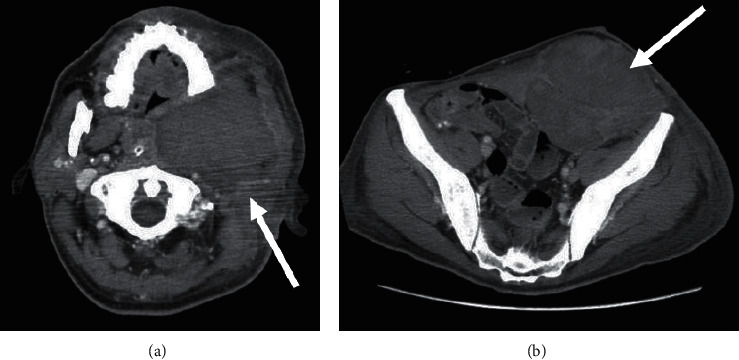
Computed tomography showed massive subcutaneous hemorrhage in the left neck (a) and abdomen (b) (arrows).
